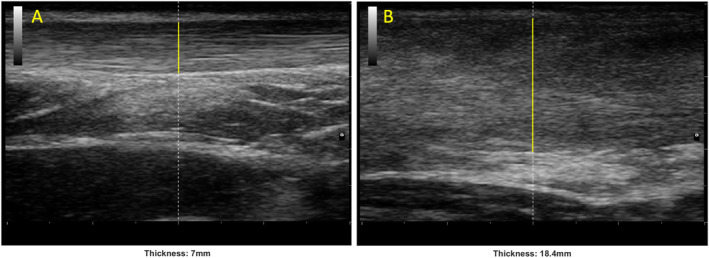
Ultrasound images representing the thickness of the Achilles tendon in a longitudinal scan. (A) Unaffected side and (B) affected side.
